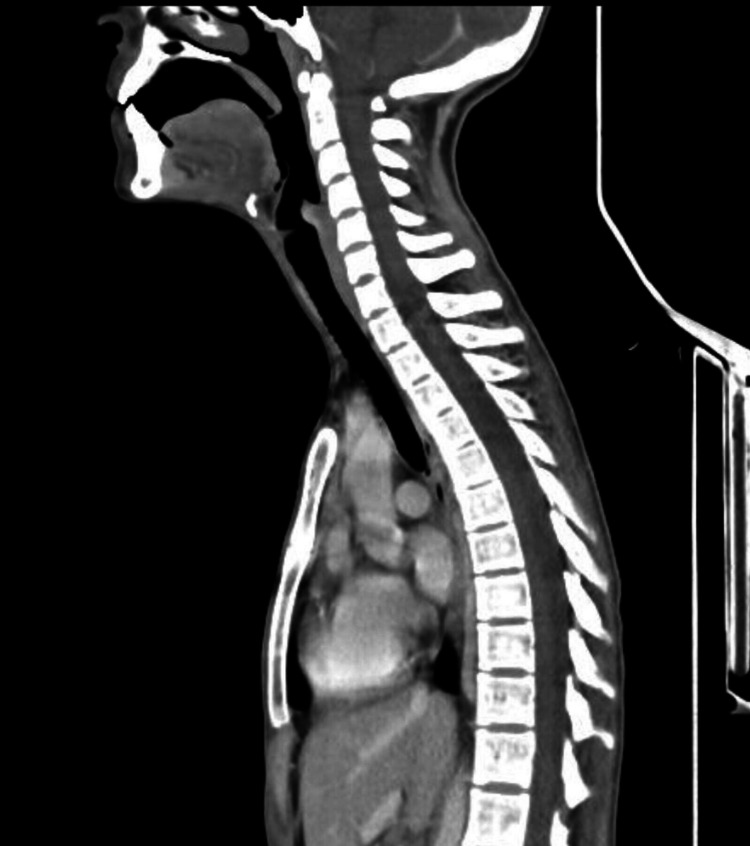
CT scan of neck and chest shows no evidence of retropharyngeal air or mediastinal emphysema.
